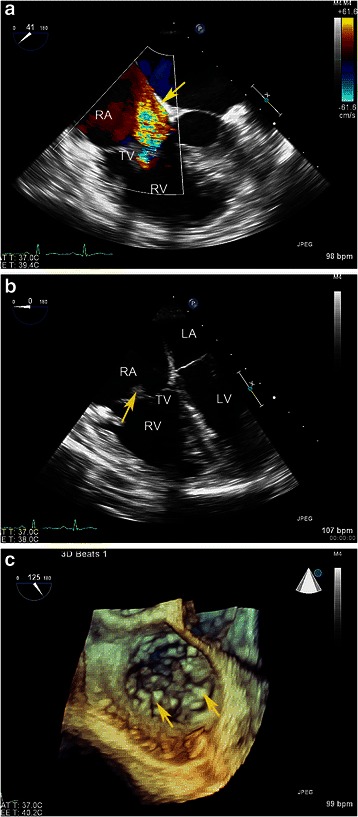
Transesophgeal echocardiography images of the patient before surgery. a Tricuspid regurgitation, Yellow arrowhead: wide and reversed blood flow signals at TV site. b A large vegetation formation. Yellow arrowhead: A large vegetation adhere to anterior leaflet of TV. c Suspicious multiple vegetations on 3D echo image. Yellow arrowheads: multiple verrucous abnormal nodular projections on the leaflet surface. *RA* right atrium, *RV* right ventricle, *LA* left atrium, *LV* left ventricle, *TV* tricuspid valve
